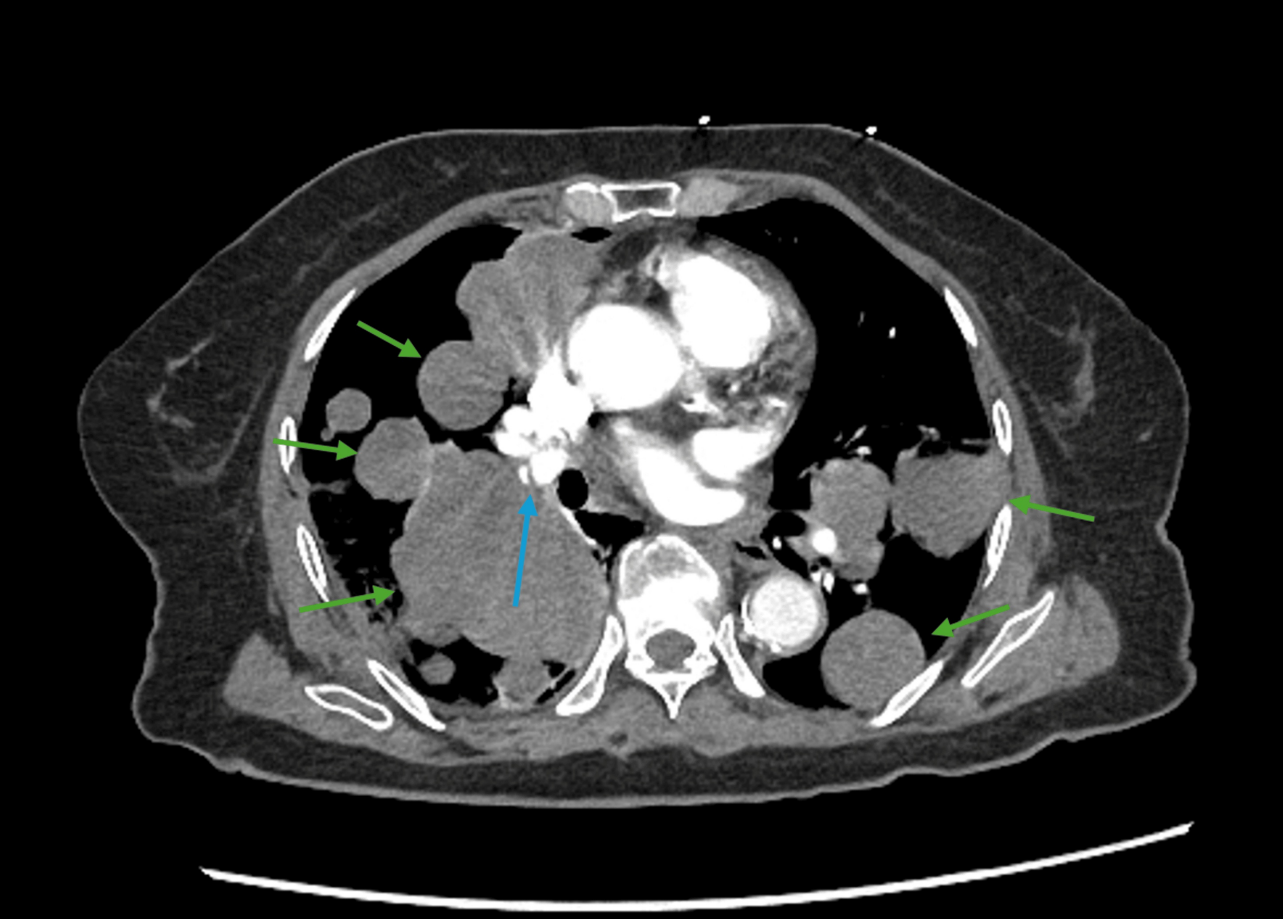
CT angiography of the chest with contrast The blue arrow points at a linear filling defect in the right lower lobe pulmonary artery consistent with acute pulmonary embolus. The green arrow points at multiple bilateral large soft tissue densities, indicating metastasis. The right lower lobe airspace is obscured by mass.
